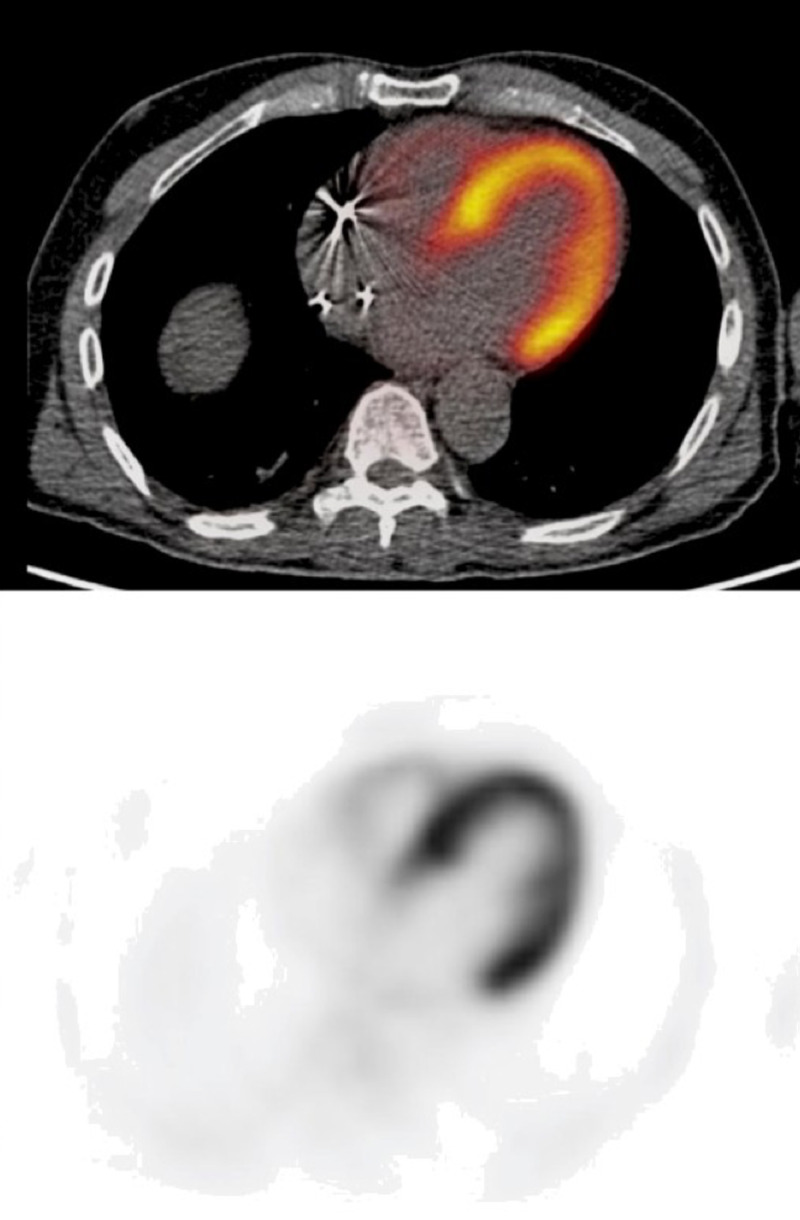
18F-FDG PET scan revealing normal perfusion at baseline without abnormal uptake suggesting resolution of cardiac sarcoid involvement 18F-FDG - 18F-fluorodeoxyglucose
